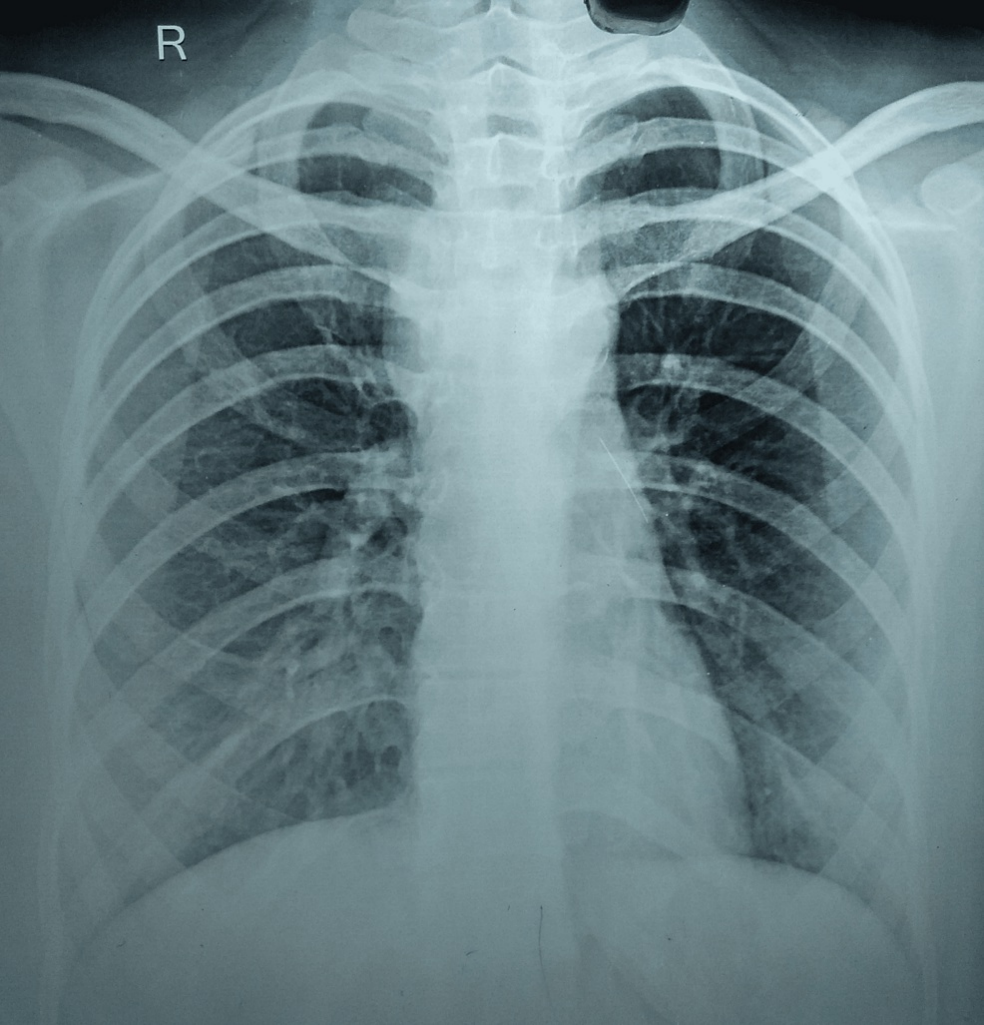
A plain radiograph of the chest not suggestive of pulmonary tuberculosis
